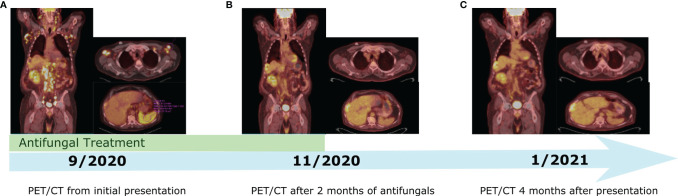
Timeline of PET/CT imaging throughout the patient's course. (A) PET/CT from Initial presentation with fever and diffuse FDG avid lymphadenopathy. (B) PET/CT showing significant decrease in size and metabolic activity of previously enlarged lymph nodes after two months of antifungal treatment. (C) PET/CT showing complete resolution of lymphadenopathy 4 months after initial presentation.
